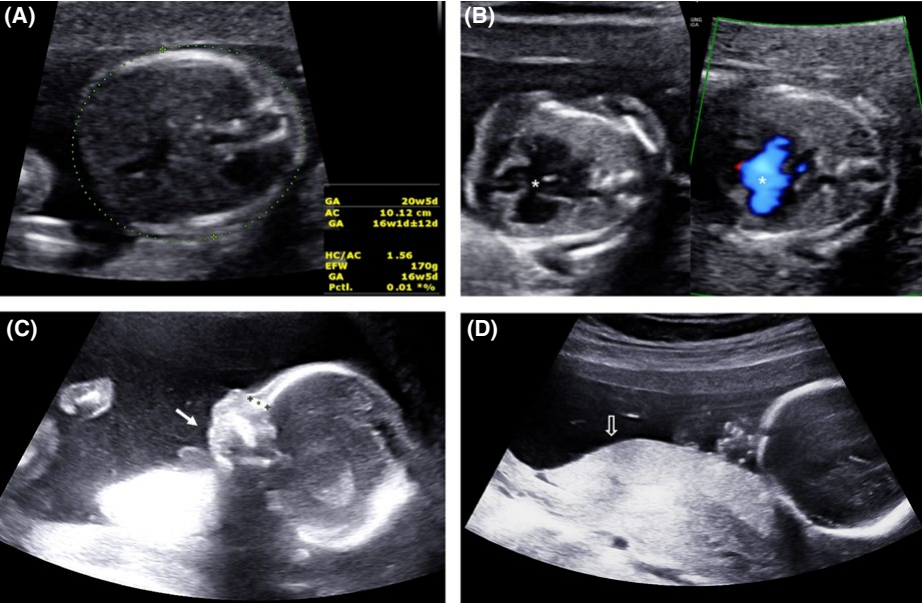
Ultrasonographic findings at 20 wk, 5 d of gestation. A, An abdominal circumference smaller by 4 wk of gestational age. B, A complete atrioventricular canal defect (asterisk). C, Micrognathia (arrow). D, Placenta (open arrow)
